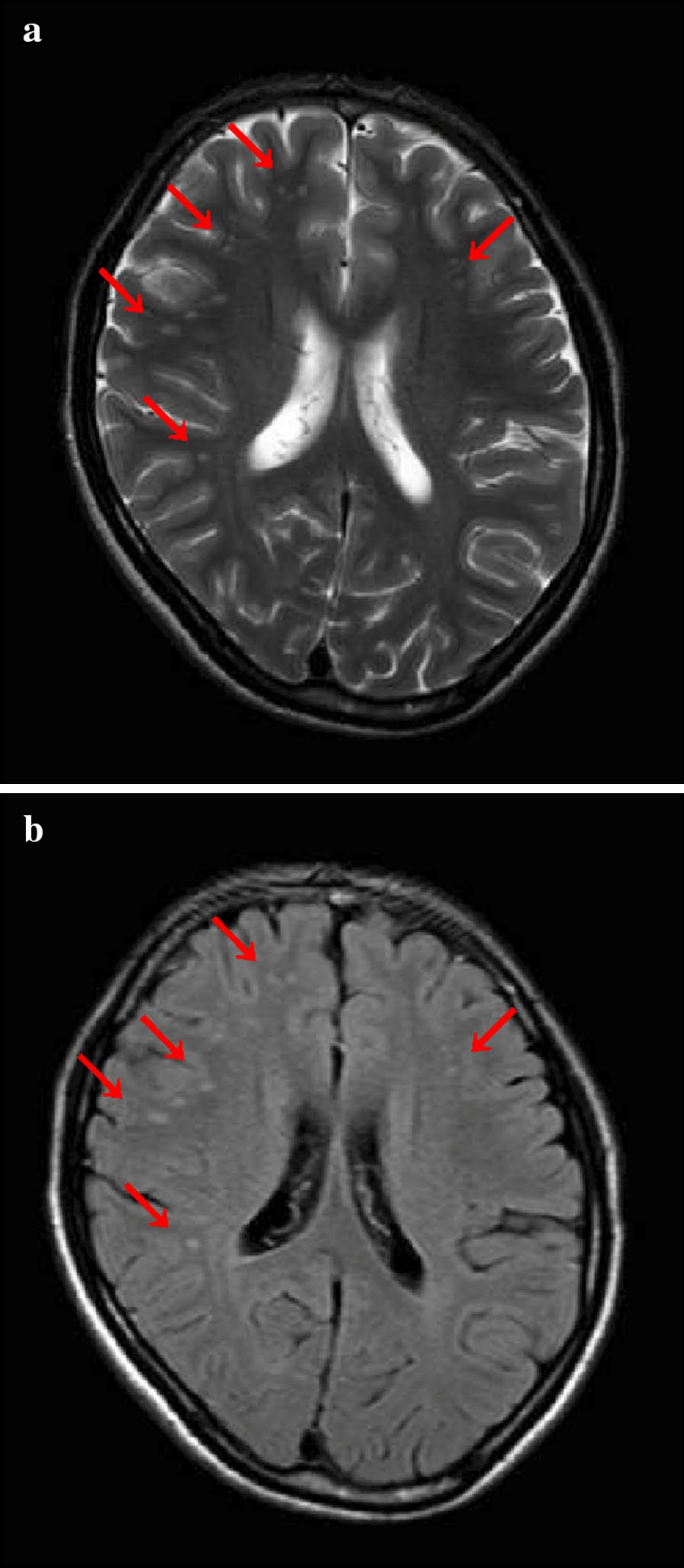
Magnetic resonance imaging (MRI) of the brain after hospitalization: a T2-weighted image. b Fluid-attenuated inversion recovery (FLAIR) image
